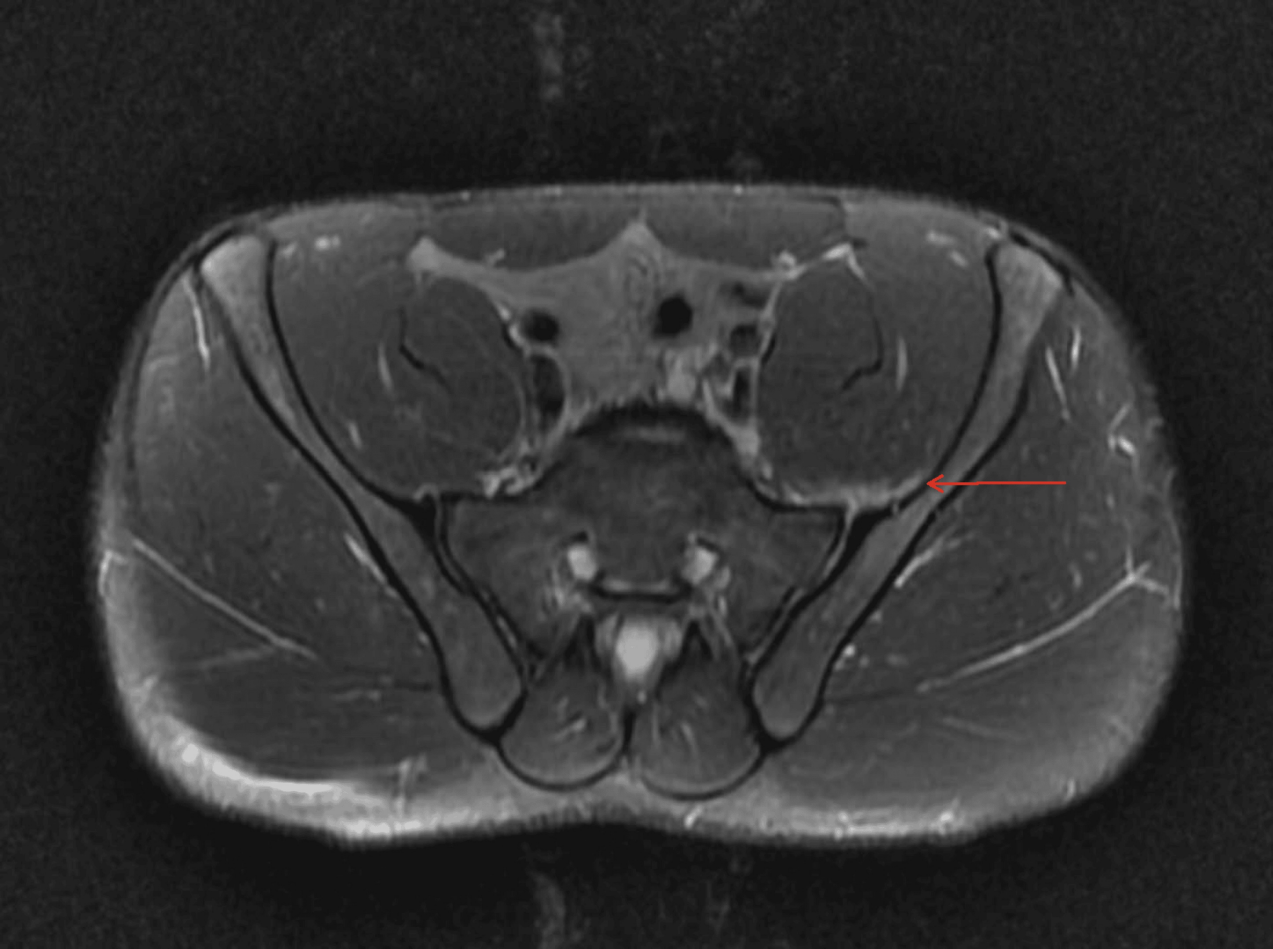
Axial T2-weighted MRI of the pelvis, with and without contrast, demonstrating soft tissue edema anterior to the left sacroiliac joint along the deep fibers of the iliopsoas muscle. The red arrow indicates the site of pathology.
